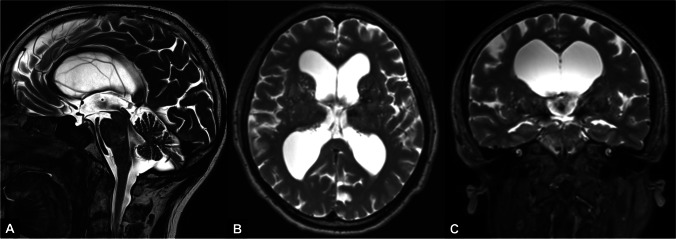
T2-weighted MRI showing landmark features of LOVA in midsagittal (A), axial (B) and coronal (C) view. A Empty sella, patent aqueduct with turbulent flow and an enlarged cisterna magna are reported. B, C Concomitant severe supratentorial ventriculomegaly is observed. Evans Index: 0.47. Third ventricle width: 26 mm
